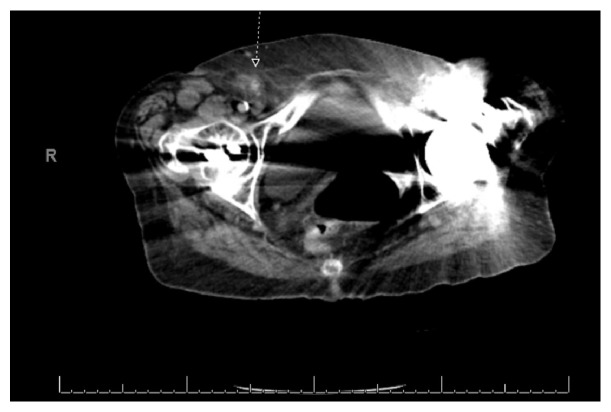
CT abdomen/pelvis image of Amyand's hernia is referenced by arrow.
